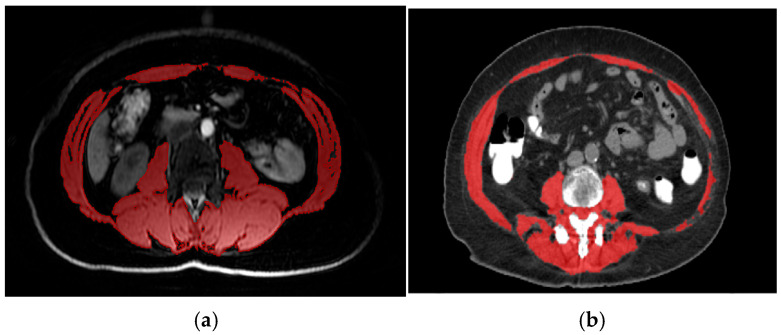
(a) Axial PET-MRI slice at the L3 vertebra in a patient with triple-negative breast cancer. Skeletal muscle area (red) was manually segmented on T1-weighted, water-only images using 3D Slicer (version 5.7.0; www.slicer.org, accessed on 13 May 2024; The Slicer Community, Brigham and Women’s Hospital, Boston, MA, USA) based on anatomical landmarks and signal intensity. SMI was calculated by dividing the muscle cross-sectional area (cm^2^) by height squared (m^2^). (b) Axial PET-CT slice at the L3 vertebra in a patient with triple-negative breast cancer. Skeletal muscle area (red) was segmented using CoreSlicer (version 1.0.0; Montreal, QC, Canada) with predefined Hounsfield unit thresholds (–29 to +150 HU). SMI was calculated as total skeletal muscle area (cm^2^) divided by height squared (m^2^). White areas in both images represent contrast enhancement.
